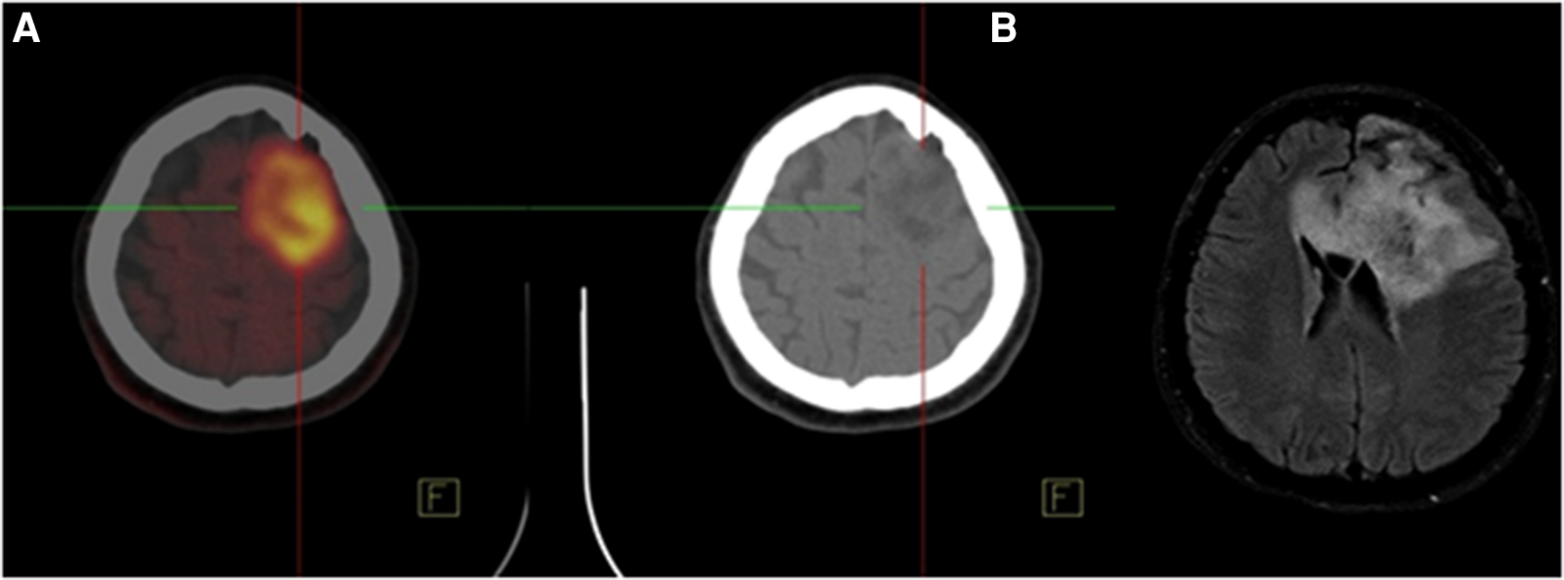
A 58-year-old man with high-grade glioma underwent brain ^18^F-FET PET/CT indicated intense radiotracer uptake (SUVmax = 7.68) in the left parietal lobe. (A) Accordingly, the tumor showed no change in Gd enhancement on FLAIR axial MRI with Gd. (B) Therefore, recurrence or residual disease was noted in the left parietal lobe.
